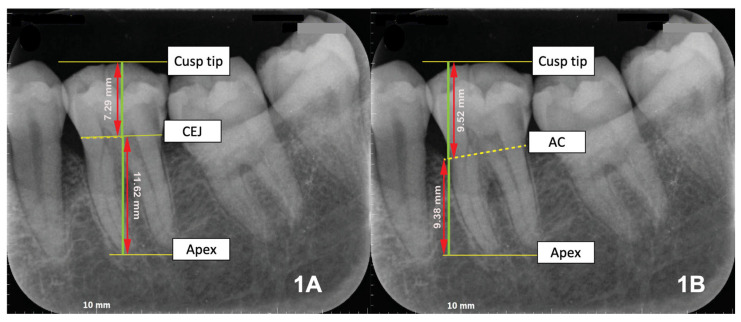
Clinical and Radiographic Crown–Root Ratios (CRR). (A) Clinical CRR: ratio of crown length (cusp tip–cemento-enamel junction [CEJ]) to root length (CEJ–apex), representing anatomical proportion. (B) Radiographic CRR: ratio of crown height (cusp tip–alveolar crest [AC]) to root length (AC–apex), reflecting remaining periodontal support. CEJ = cemento-enamel junction; AC = alveolar crest.
